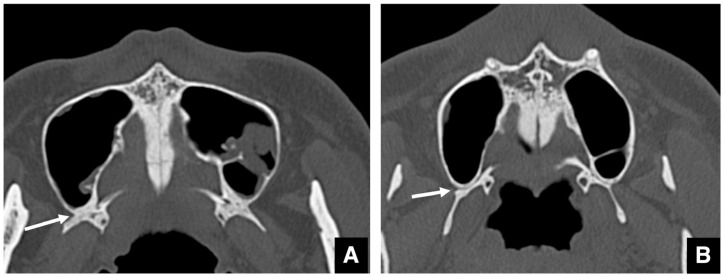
MDCT images (slice thickness, 3.0 mm) of the pterygomaxillary suture. (A) Axial bone window MDCT image shows a robust pterygomaxillary suture (arrow). Mucosal cysts are seen incidentally in the left maxillary sinus. (B) Axial bone window CT image shows a fragile pterygomaxillary suture (arrow). Permission has been obtained to publish all figures.
